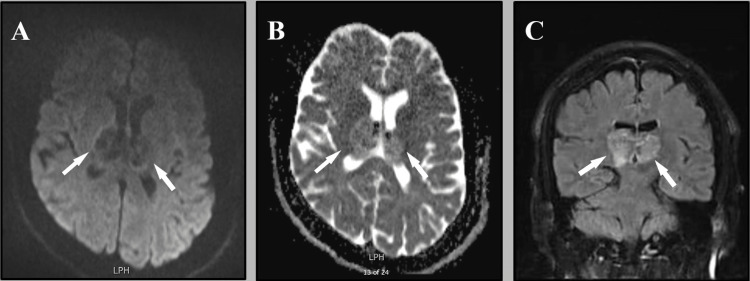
Axial MRI reveals bilateral thalamic edema, which appears as dark in DWI (white arrows) (A), and white in ADC (white arrows) (B). Coronal MRI FLAIR showing bithalamic hyperintense lesion (white arrows) (C).
